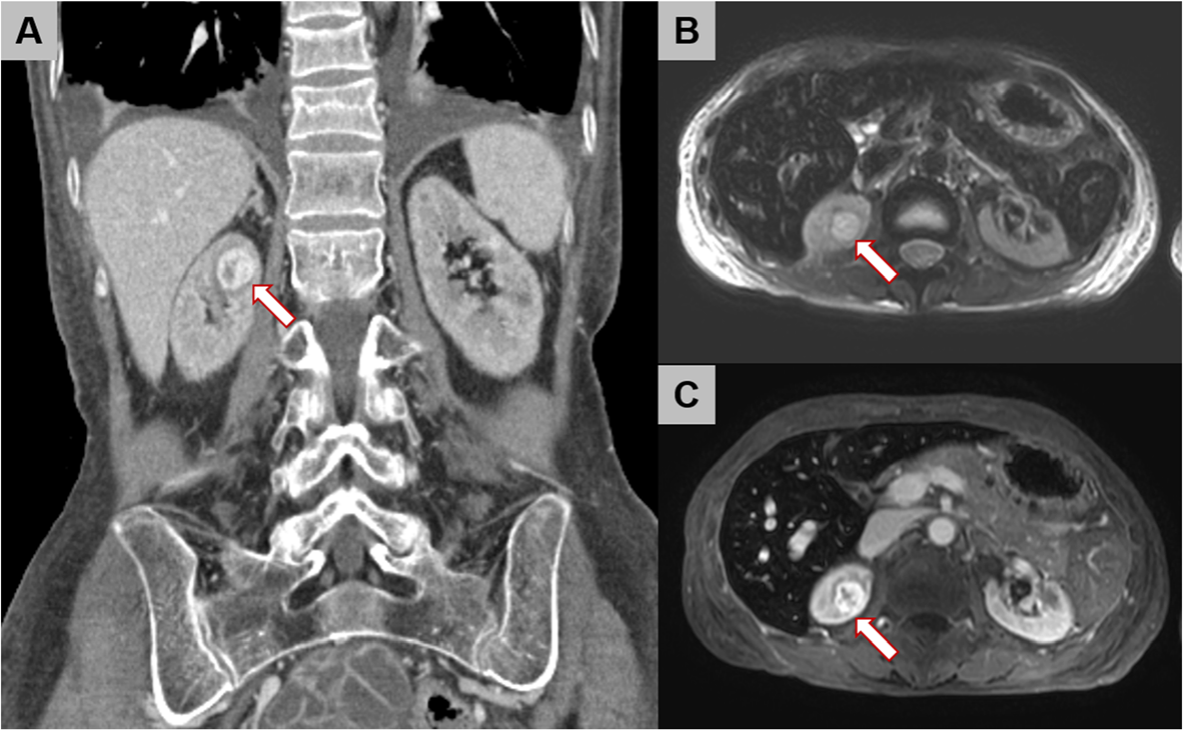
A Contrast-enhanced computed tomography scan revealed the heterogeneously enhancing mass in the right kidney (arrow). Kidney magnetic resonance imaging revealed the B lesion was a high signal intensity in T2-weighted image, and C showed heterogeneous enhancement (arrow)
